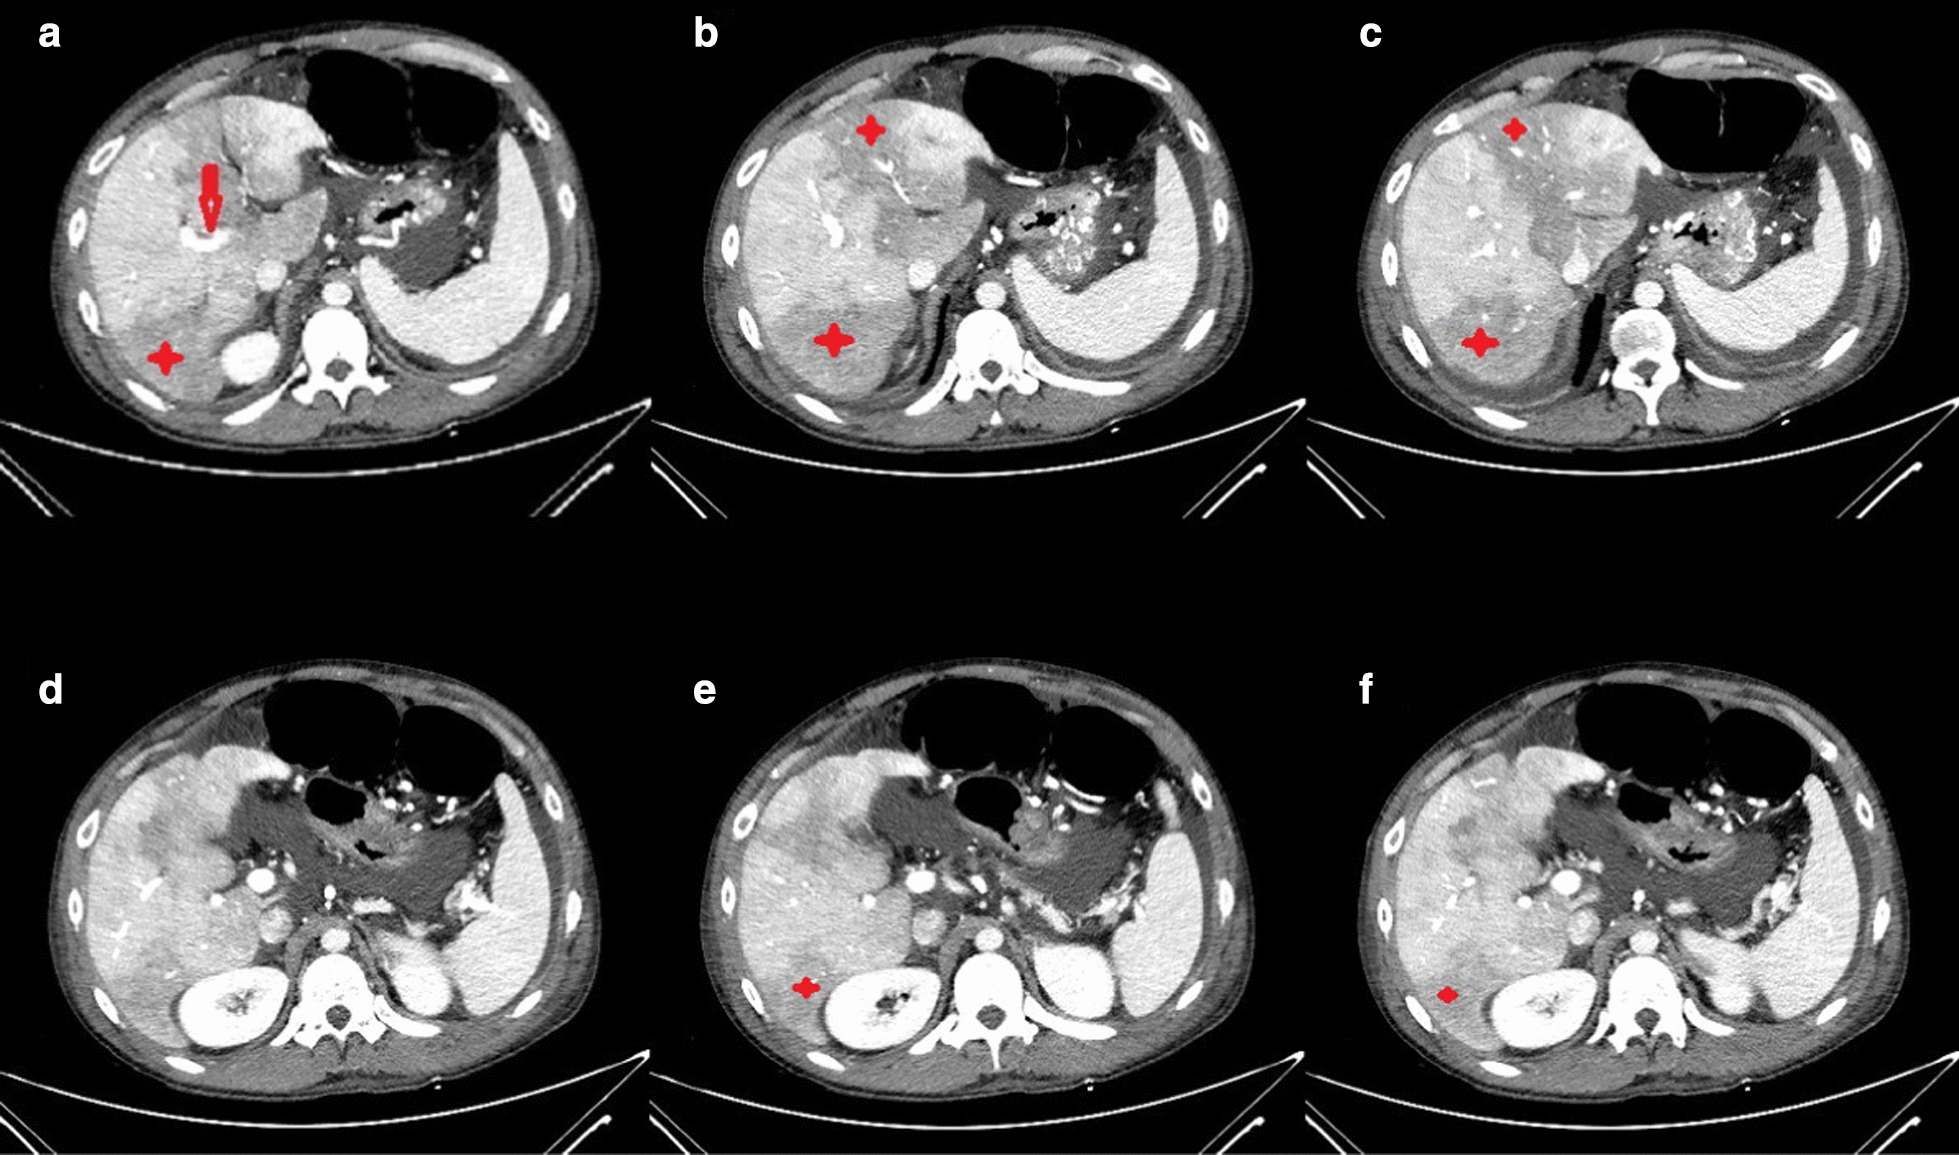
Abdominopelvic CT with IV contast. Hypoperfusion is seen in the posterior segment of right (Rt.) hepatic lobe, and the medial and lateral segments of left (Lt.) lobe (stars). Evidence of filling defect in main Rt. portal vein is also detected (arrow)
